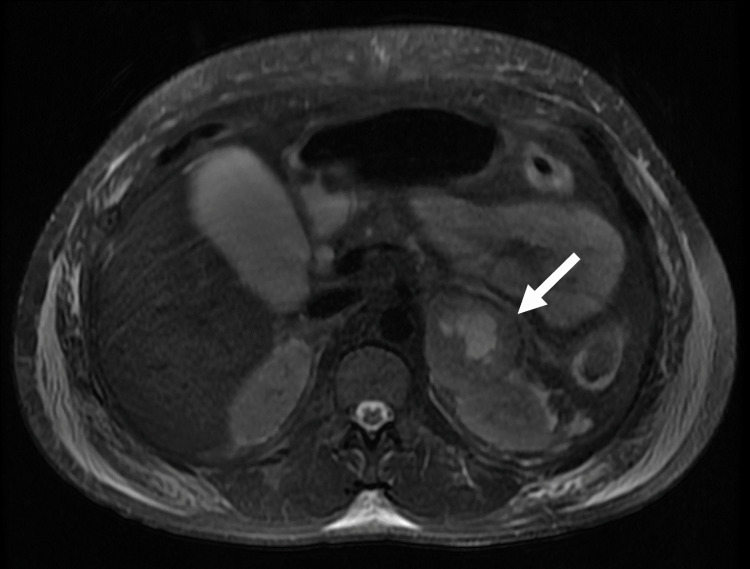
MRI T2-weighted image. Arrow pointing at the left adrenal lesion.
